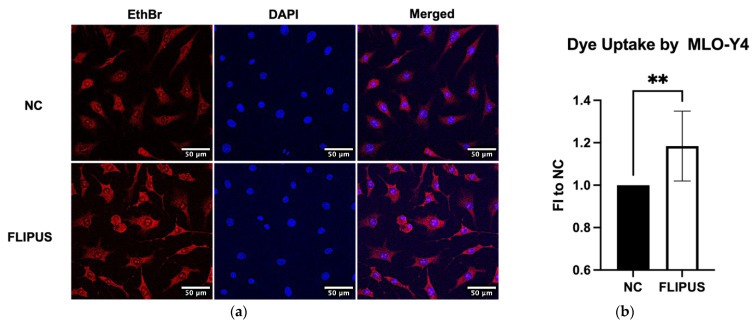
Ten-minute FLIPUS exposure of starved MLO-Y4 osteocytes enhances dye uptake by the cells. (a) Representative images of the ethidium bromide (EthBr) dye uptake; (b) Quantification results of the dye uptake assay. FI to NC stands for fold induction over negative control, which did not receive ultrasound treatment. Each data point was the result of N = 3 and n = 2, where N and n are biological trials and technical replicates, respectively. Five random pictures were analyzed for each data point.  represents *p* ≤ 0.01.
